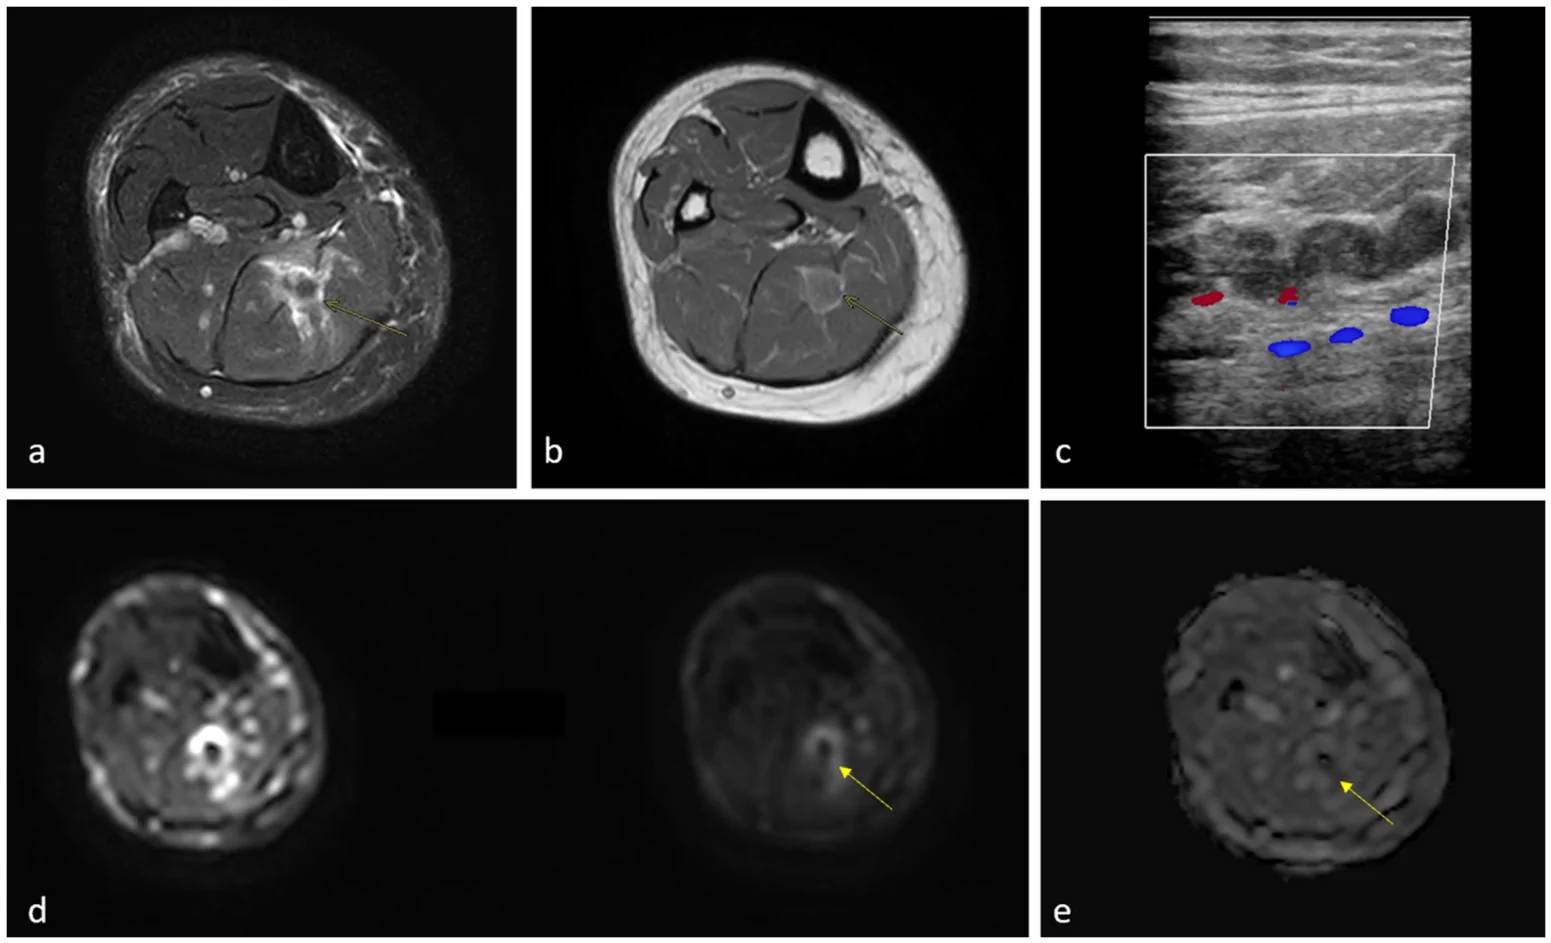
Axial MRI demonstrating soleal vein thrombosis in a patient with a sports injury suspected of a muscle tear. T2-weighted imaging shows persistent expansion of the soleal vein with hypointense intraluminal signal (a) and hyperintense signal on the T1-weighted image with luminal expansion (b), with correspondingly low signal on DWI and the ADC map (d,e) (yellow arrows), in keeping with non-acute (subacute) thrombosis (image-based diagnosis only). Targeted colour Doppler ultrasound (white box) showed no colour flow confirming the diagnosis (c). This demonstrates the diagnostic value of DWI in the detection of deep vein thrombosis.
